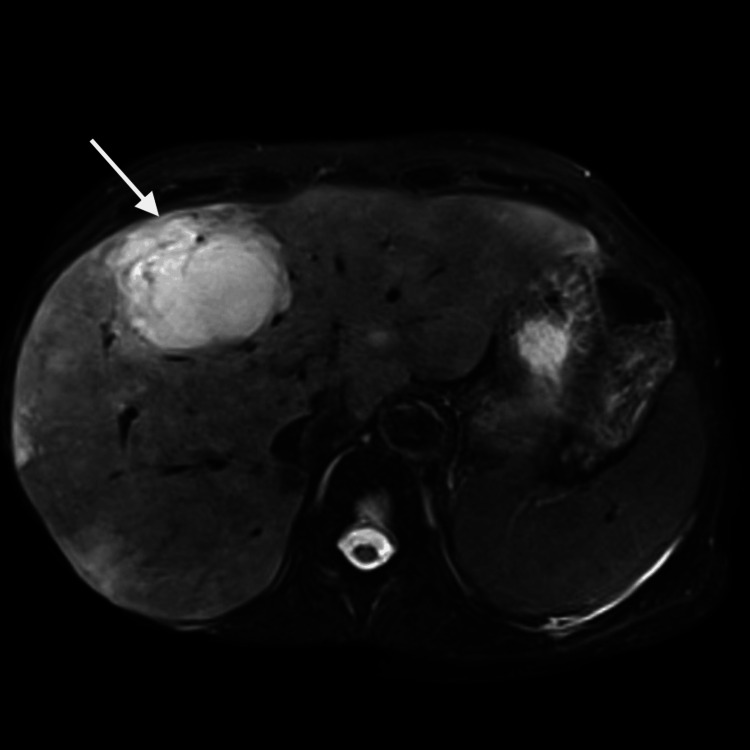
Axial T2-weighted MRI of the upper abdomen demonstrating hepatomegaly, approximately 18.2 cm in longitudinal diameter, with lobulated contours and markedly heterogenous signal intensity dysplaing a mottled appearance and several ill-defined hypoenchancing foci. A thin, filiform filling defect is seen in the central lumen of the right hepatic vein, along with significant narrowing of the retrohepatic inferior vena cava at its uppermost segment.
